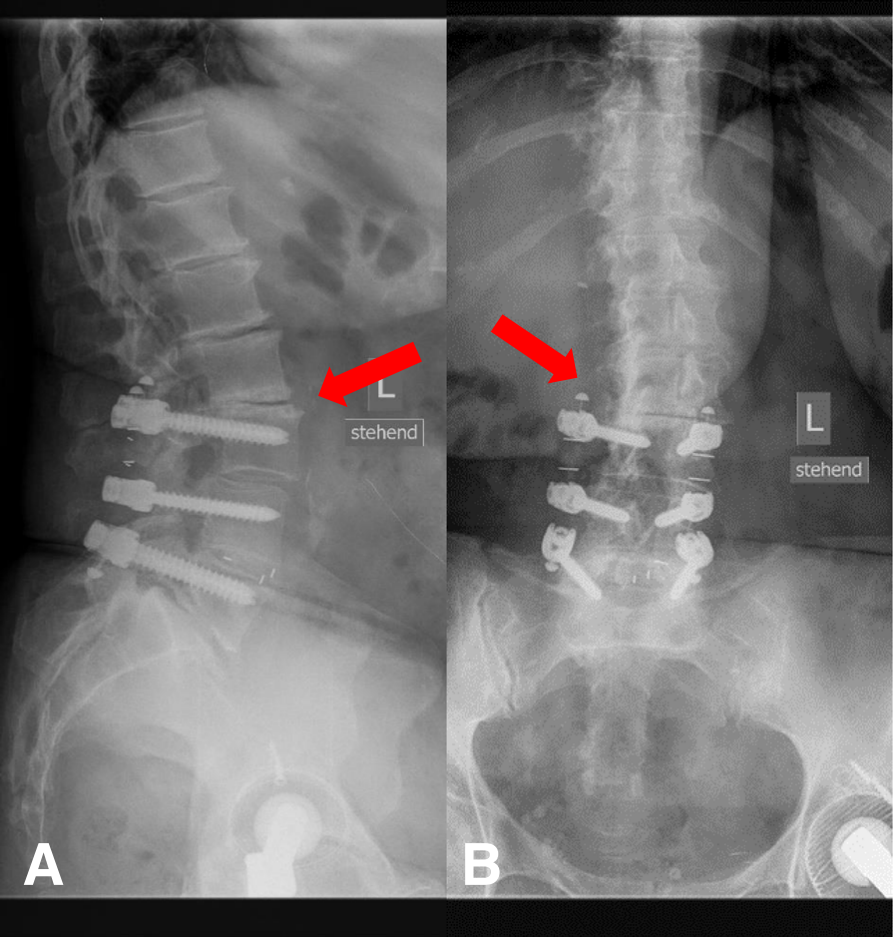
Lumbar spine lateral (a) and anterior–posterior (b) radiographs at 2 years after surgery. Radiological signs of degeneration (red marker) in the segment cranially adjacent to the topping off segment
